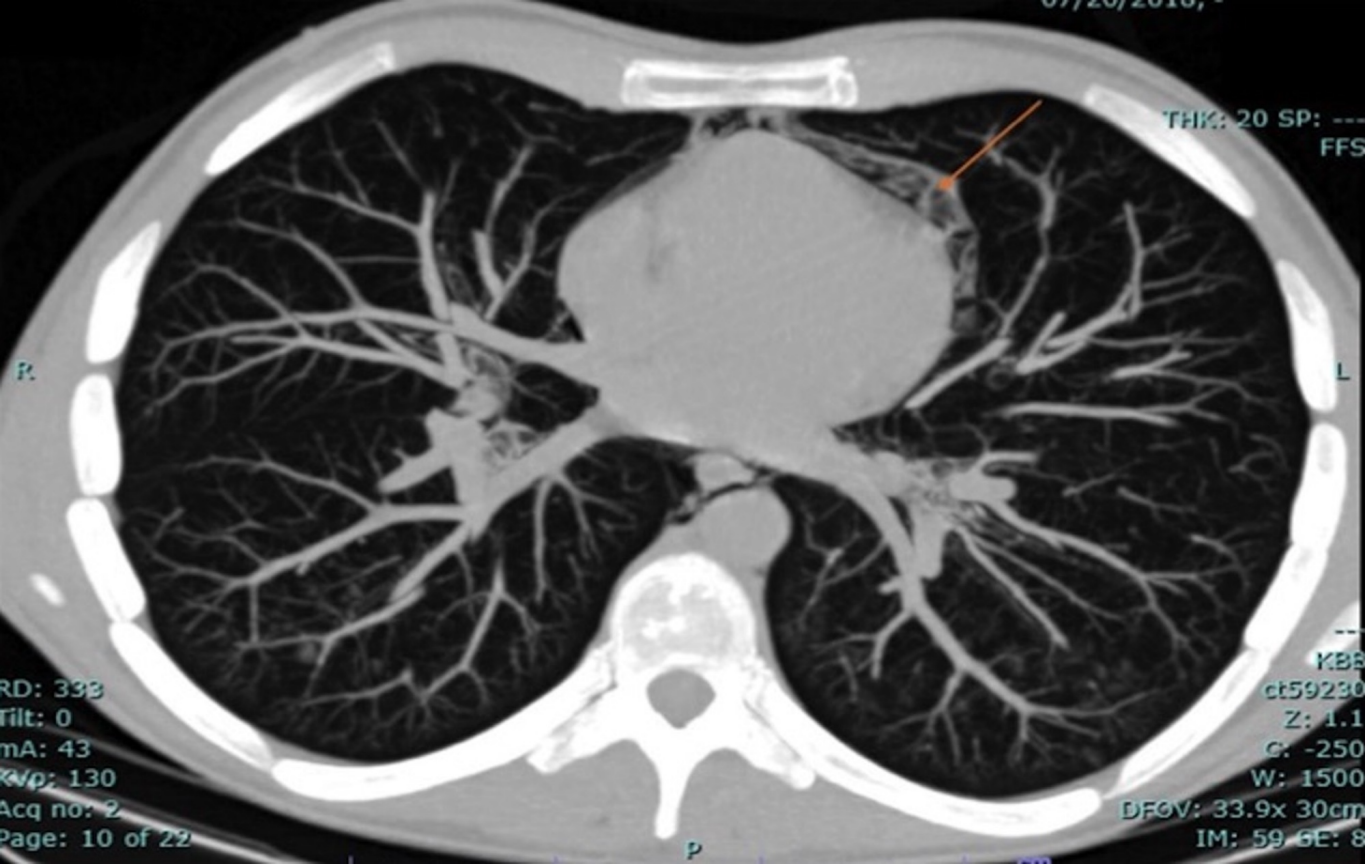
Computed tomography of the chest demonstrating pneumopericardium.
